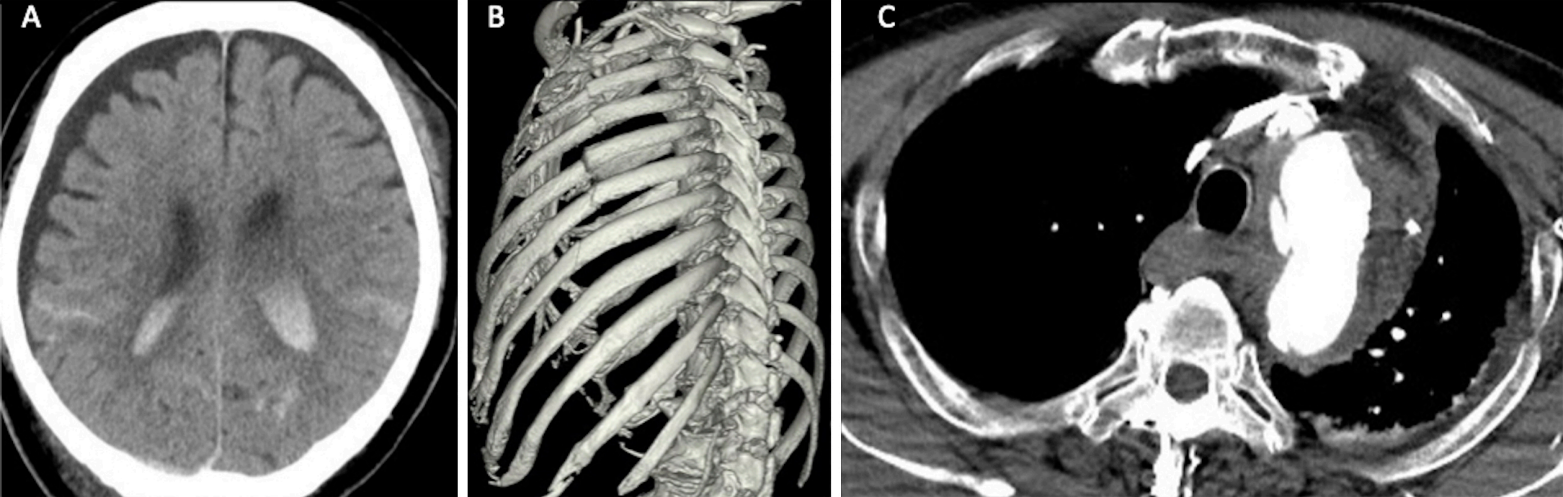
Computed tomography revealed intracranial injuries (A), multiple rib fractures (B), and aortic arch pseudoaneurysm (C).
